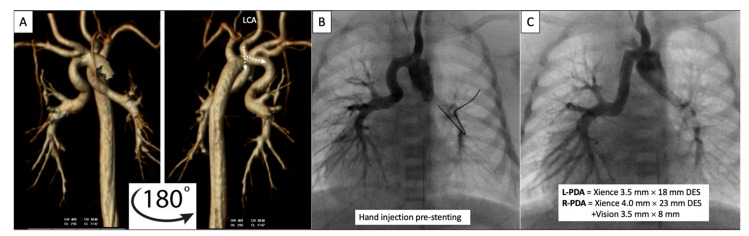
Pre-procedural 3D virtual CT imaging (A) predicts the left carotid artery (LCA) as the best access site for bilateral PDA stenting (B,C) in a patient with discontinuous PAs. Multi-Link Vision and Xience Alpine^TM^ drug eluting (DES) stents (Abbott Vascular Company, Abbott Park, IL, USA) were used. Revised from ref [9].
